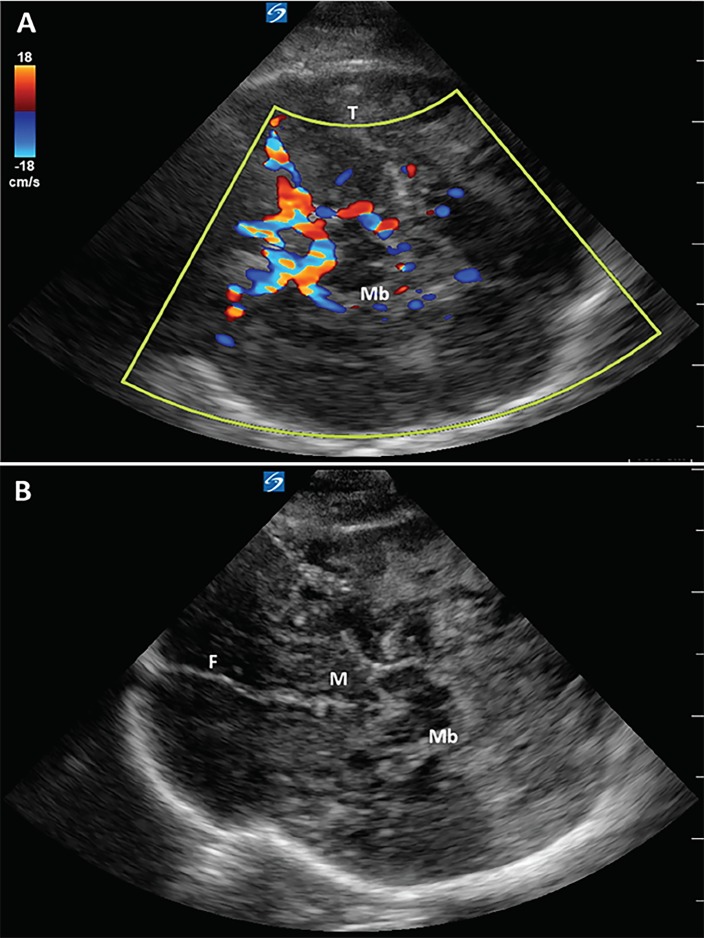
Cranial ultrasound with Doppler (panel A) and without Doppler (panel B) through the left transtemporal window in a 74-year-old male with a left hemicraniectomy with additional anatomical details. *T*, thalami; *Mb*, midbrain; *F*, falx cerebri; *M*, mammillary bodies.
